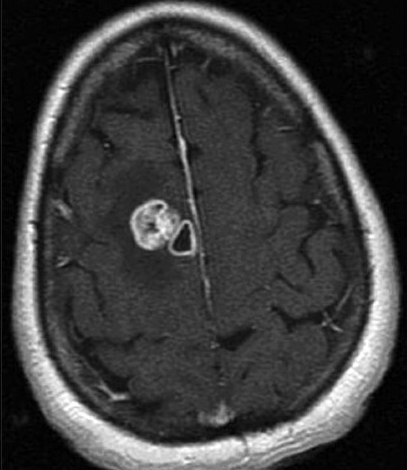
T1-weighted MR images with gadolinium. Nonhomogeneously enhancing right parietal tumor
